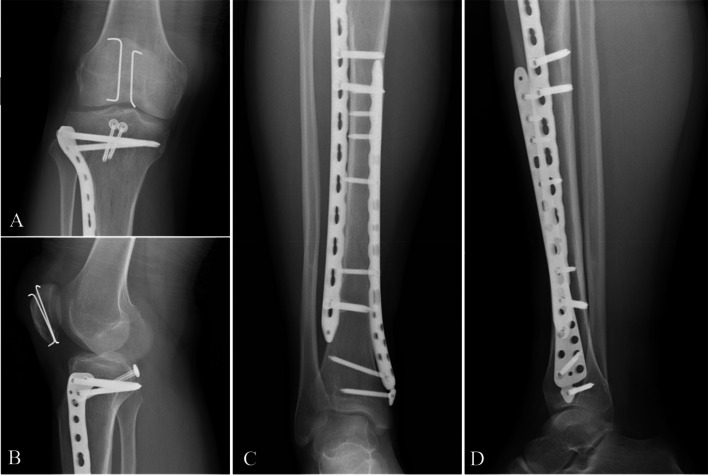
Postoperative radiographs at 3 months after surgery: AP (A) and lateral (B) views of the knee showed good healing of the PCL tibial insert, patella, and tibial plateau fractures. AP (C) and lateral (D) views of the tibia showed good healing in the tibial shaft fractures
